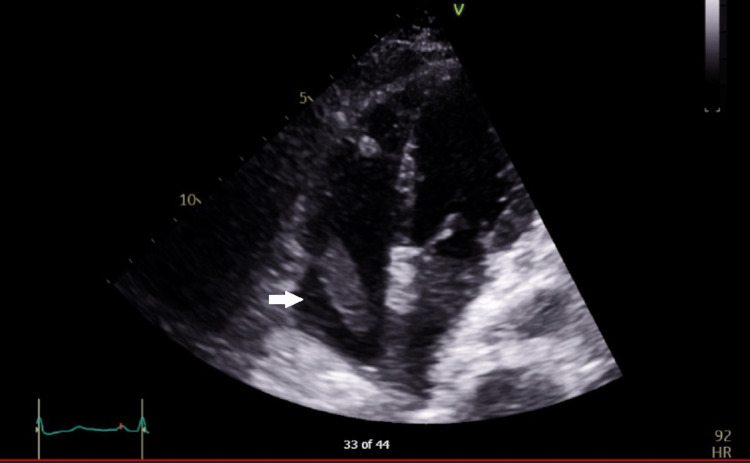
Transthoracic echocardiogram showed right atrium thrombus.
